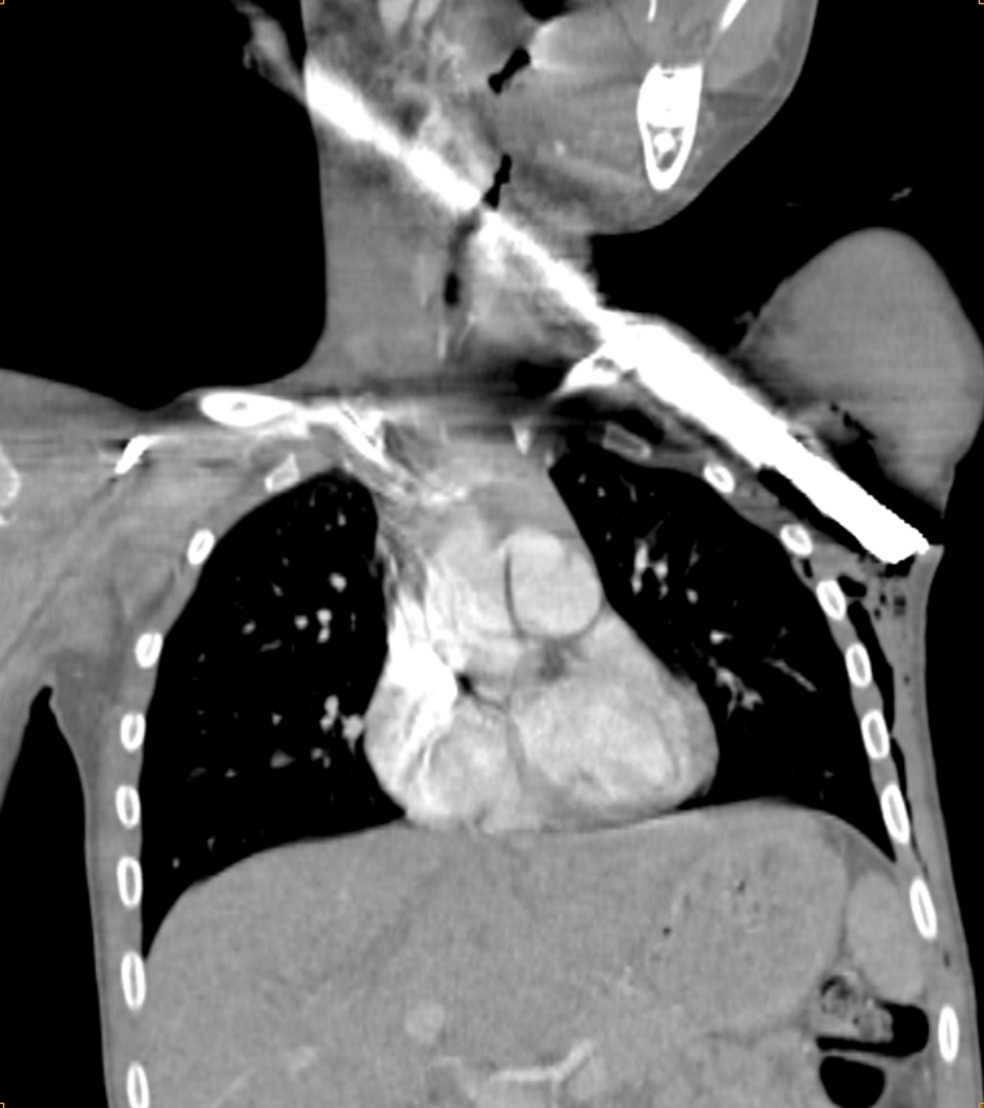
CT angiography of the chest showing the presence of subcutaneous emphysema in the soft tissues of the left posterolateral chest wall without major injuries.
